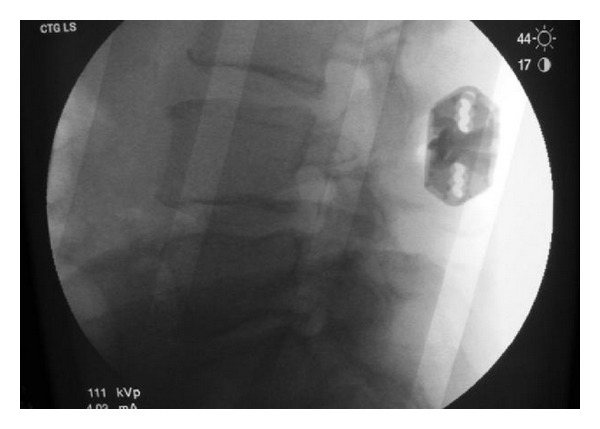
Intraoperative X-ray image of a Stabilink implanted at L3-L4 interspinous level.
